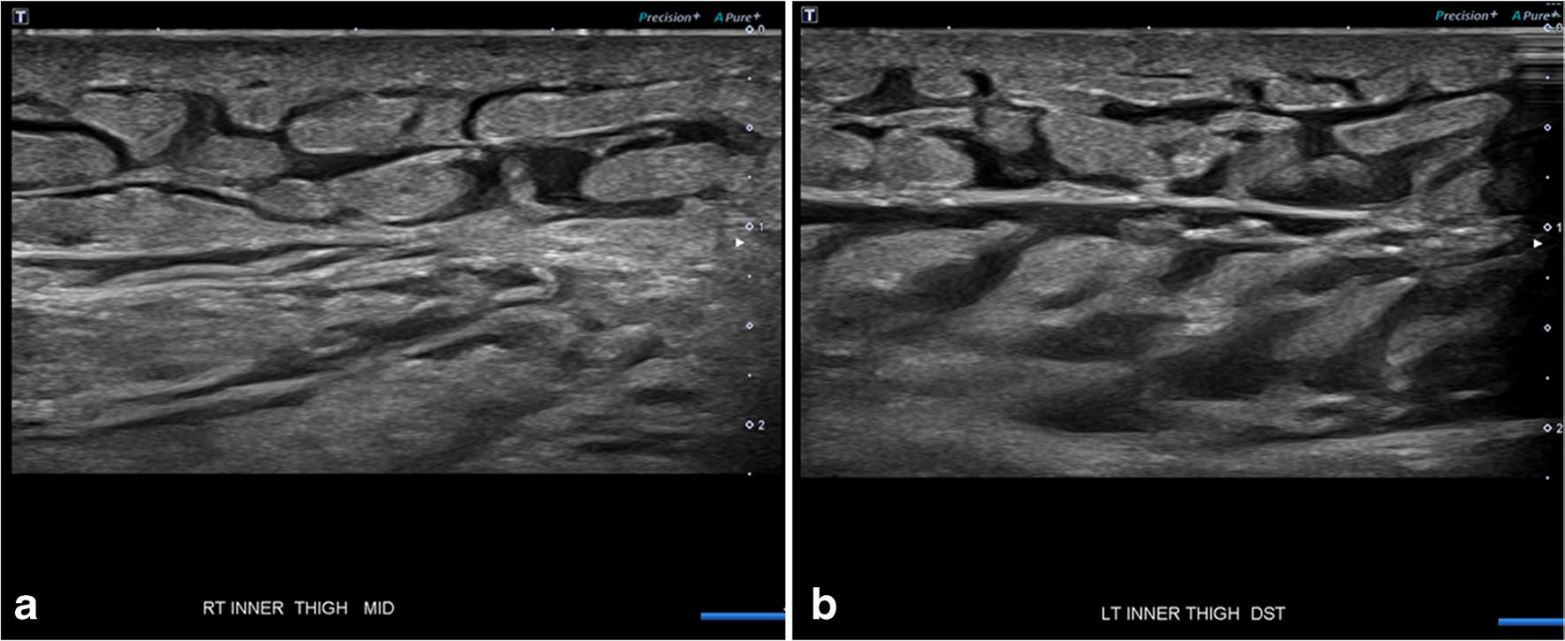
Sixty-seven-year-old man with renal failure and history of small bowel resection, total colectomy, and multiple intra-abdominal fluid collections presented with bland edema of the right (a) and left (b) upper inner thighs. Ultrasound shows “cobblestone” appearance. No focal fluid collection was seen
